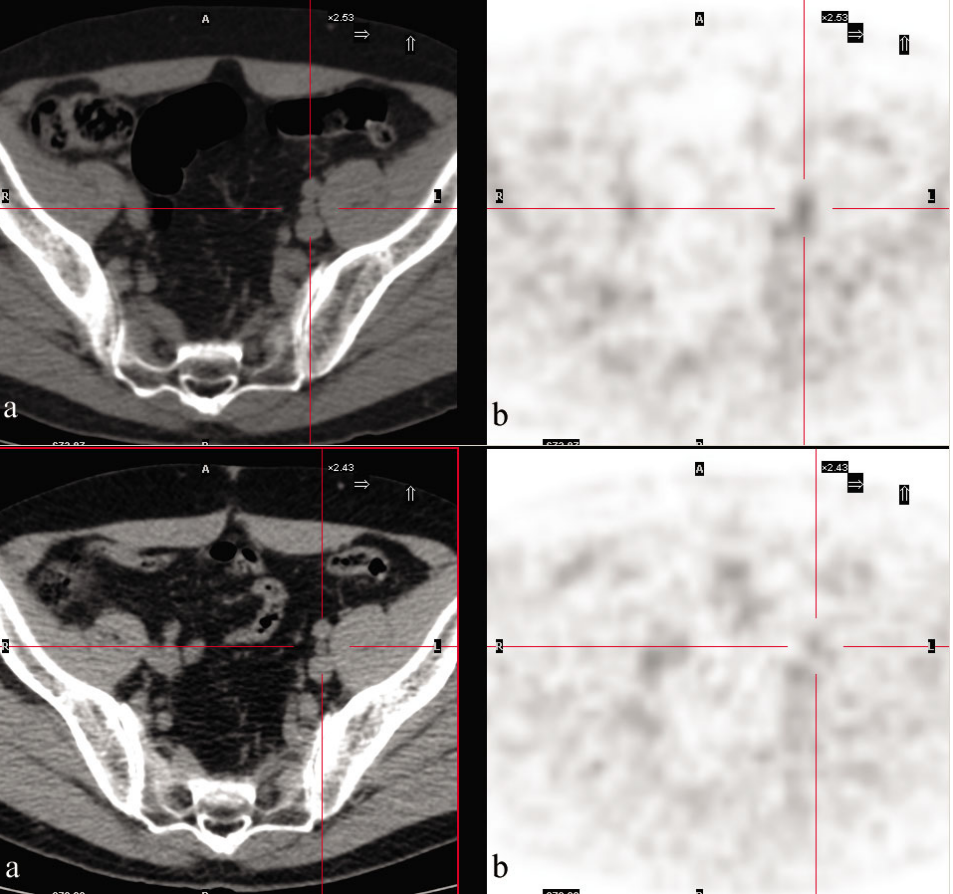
FDG-PET/CT transaxial views of the left iliac lymph node. (A) Low-dose CT; (B) PET. Upper images: pre-treatment (SUVmax 2.4); lower images: post-treatment (SUVmax 1.8).
